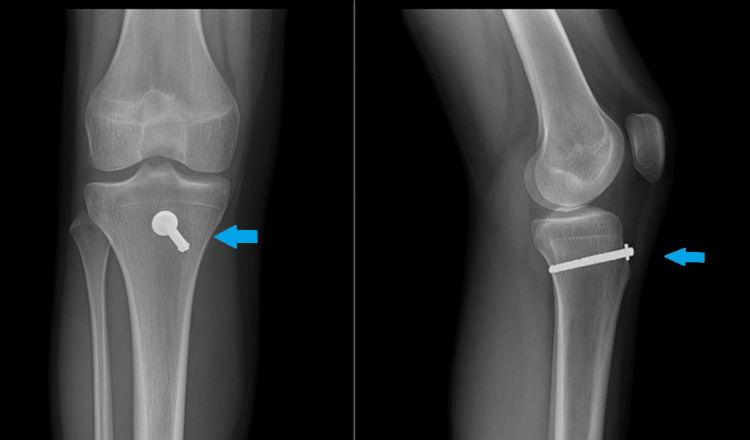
X-ray image at 10-week review. Metalwork was removed at 13 months postoperatively (blue arrow).
